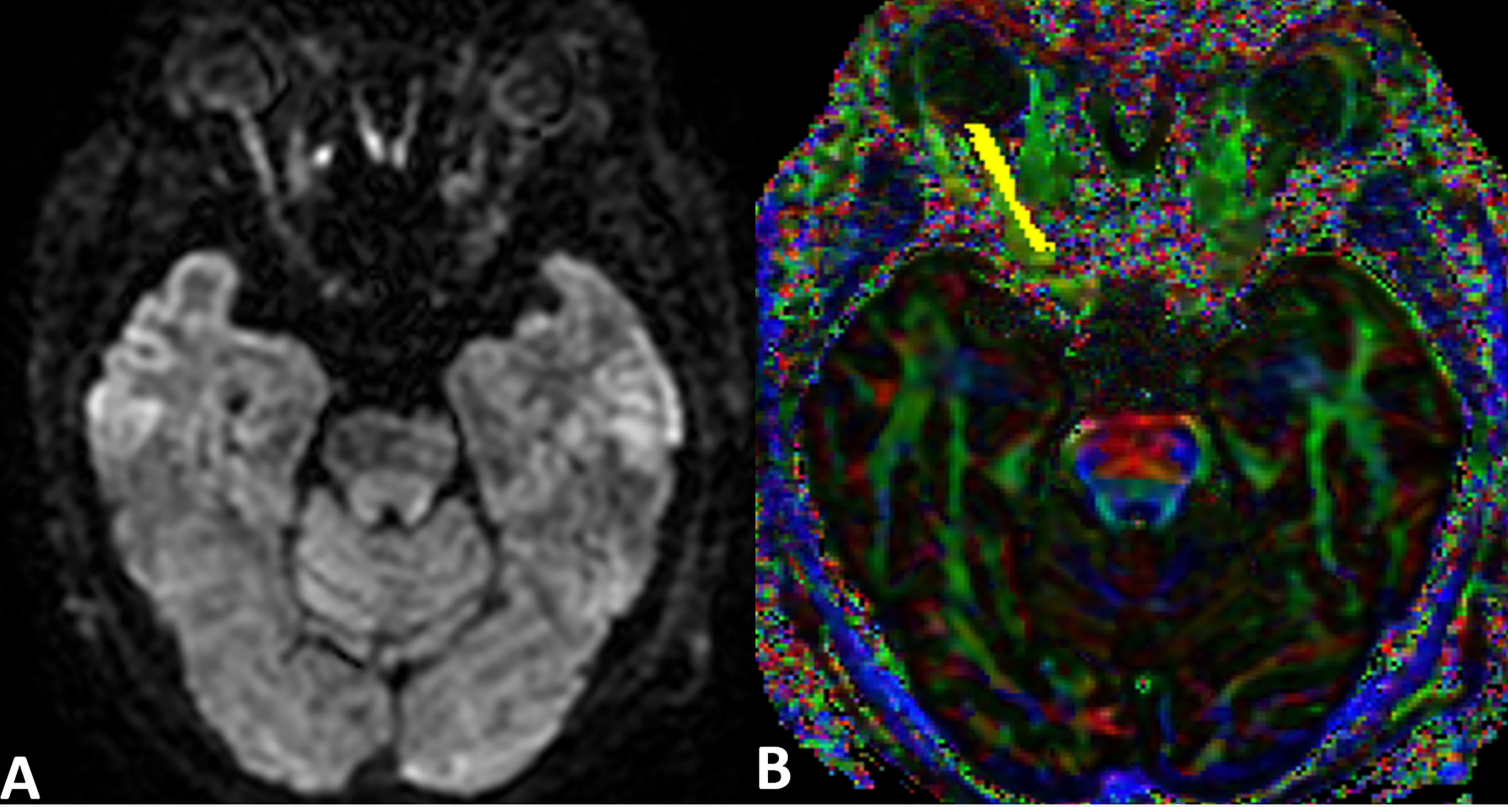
An example raw DWI image and DTI color map of the optic nerve. (A) A raw DWI image of the optic nerve. (B) A combined FA and directional color map of the optic nerve generated by MRI Studio. The region of interest is indicated in yellow. The color hue indicates direction as follows: red, left-right; green, anteroposterior; blue, superior-inferior.
